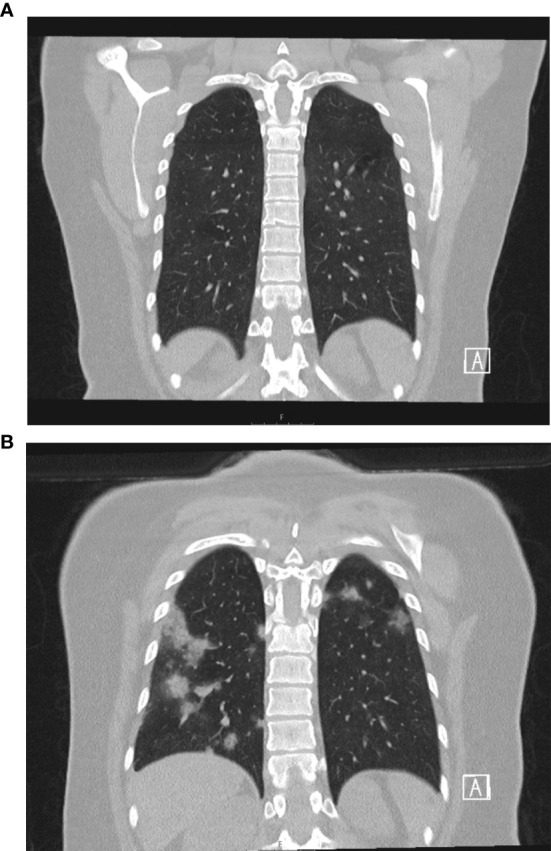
Chest CT scans on day 3 after admission (A) without signs of COVID-19 and day 34 (B) showing COVID-19 pneumonia.
